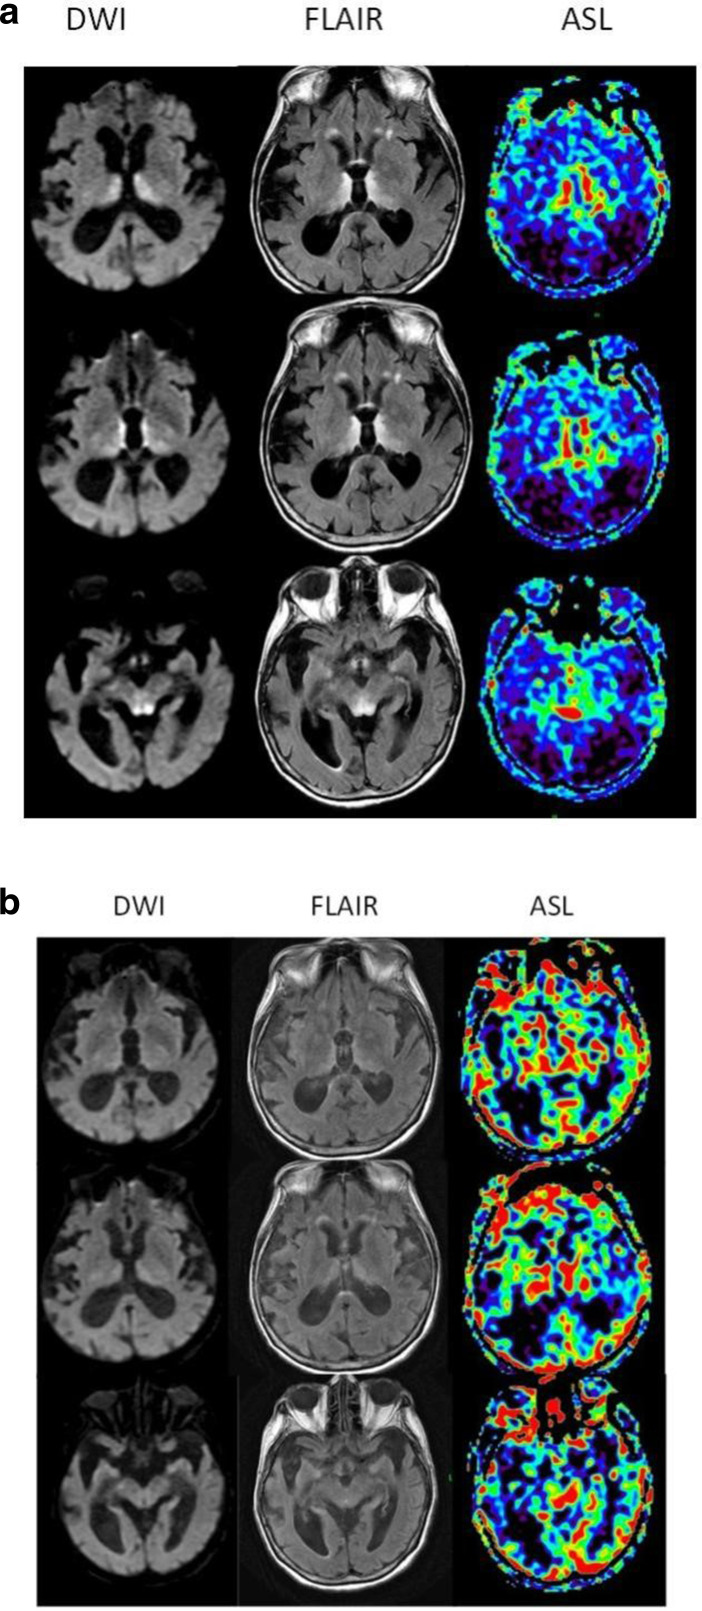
(a) Pre-treatment MRI in Patient 2 demonstrates restricted diffusion and T2 prolongation in the medial thalami, mammillothalamic tracts, and periaqueductal gray. These areas again corresponded to increased CBF on ASL, with relative cerebral cortical hypoperfusion. (b) Post-treatment MRI in Patient 2 showed improvement of restricted diffusion and T2 prolongation of the medial thalami, mammillothalamic tracts, and periaqueductal gray, along with normalization of the increased CBF previously seen on ASL. There is correction of prior global cerebral hypoperfusion.
